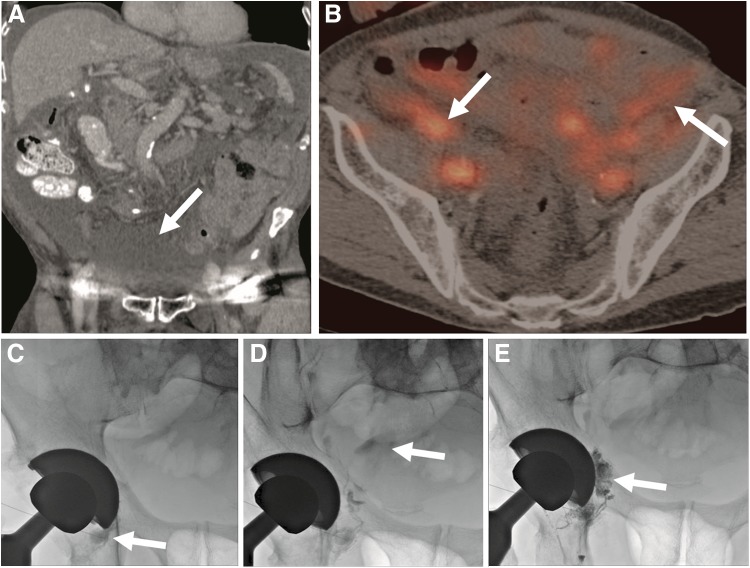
Eighty-four-year-old male with a history of muscle-invasive bladder cancer status post-cystoprostatectomy with ileal conduit. The patient presented with recurrent abdominal distension and lymphatic ascites. (A) Coronal computed tomography image of the abdomen demonstrated large volume ascites (*arrow*). (B) Nuclear medicine SPECT/CT Tc99m sulfur colloid lymphoscintigraphy demonstrated progressive pooling of radiotracer within the pelvis (*arrows*), compatible with a lymphatic leak. (C) A 25-gauge spinal needle was inserted into a right inguinal lymph node (*arrow*) and pelvic lymphangiography was performed. (D) With continued injection of contrast, extravasation was seen emanating from a right pelvic lymphatic vessel with pooling in the right hemipelvis (*arrow*). (E) The needle was subsequently primed with 5% dextrose. *n*-BCA glue was mixed with ethiodized oil (1 mL cyanoacrylate: 4 mL ethiodized oil) and was injected, thereby embolizing the leak (*arrow*). The patient's ascites subsequently resolved. CT, computed tomography; SPECT, single-photon emission computed tomography. *n*-BCA, *n*-butyl cyanoacrylate.
